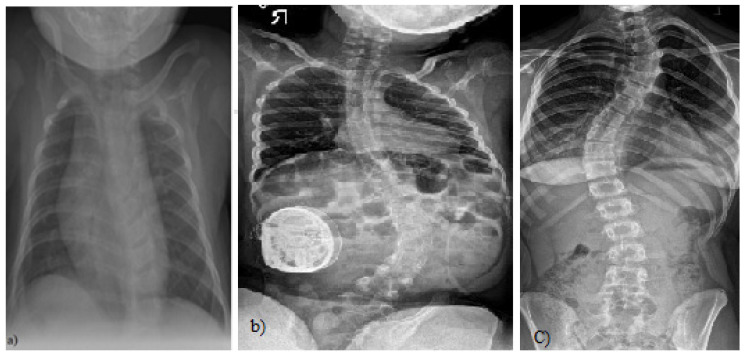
(a) A 4-month-old boy born small for gestational age, at 37 weeks, who presented initially with asymmetry of both the left and right aspects of the anterior and posterior chest and confirmed thoracolumbar scoliosis and vertebral anomalies based on plain radiography with a Cobb angle measurement of 30 degrees, (Case courtesy of Sonal Desai, Radiopedia.org, rID: 63310 under Creative Commons License (CC BY 3.0).) [5]; (b) X-rays of a girl with juvenile idiopathic scoliosis, (Case courtesy of Dr Jeremy Jones, Radiopaedia.org, rID: 89566 (CC BY 3.0).) [6]; and (c) severe left thoracic adolescent scoliosis, (Case courtesy of Dr Jeremy Jones, Radiopaedia.org, rID: 89456 (CC BY 3.0).) [7].
